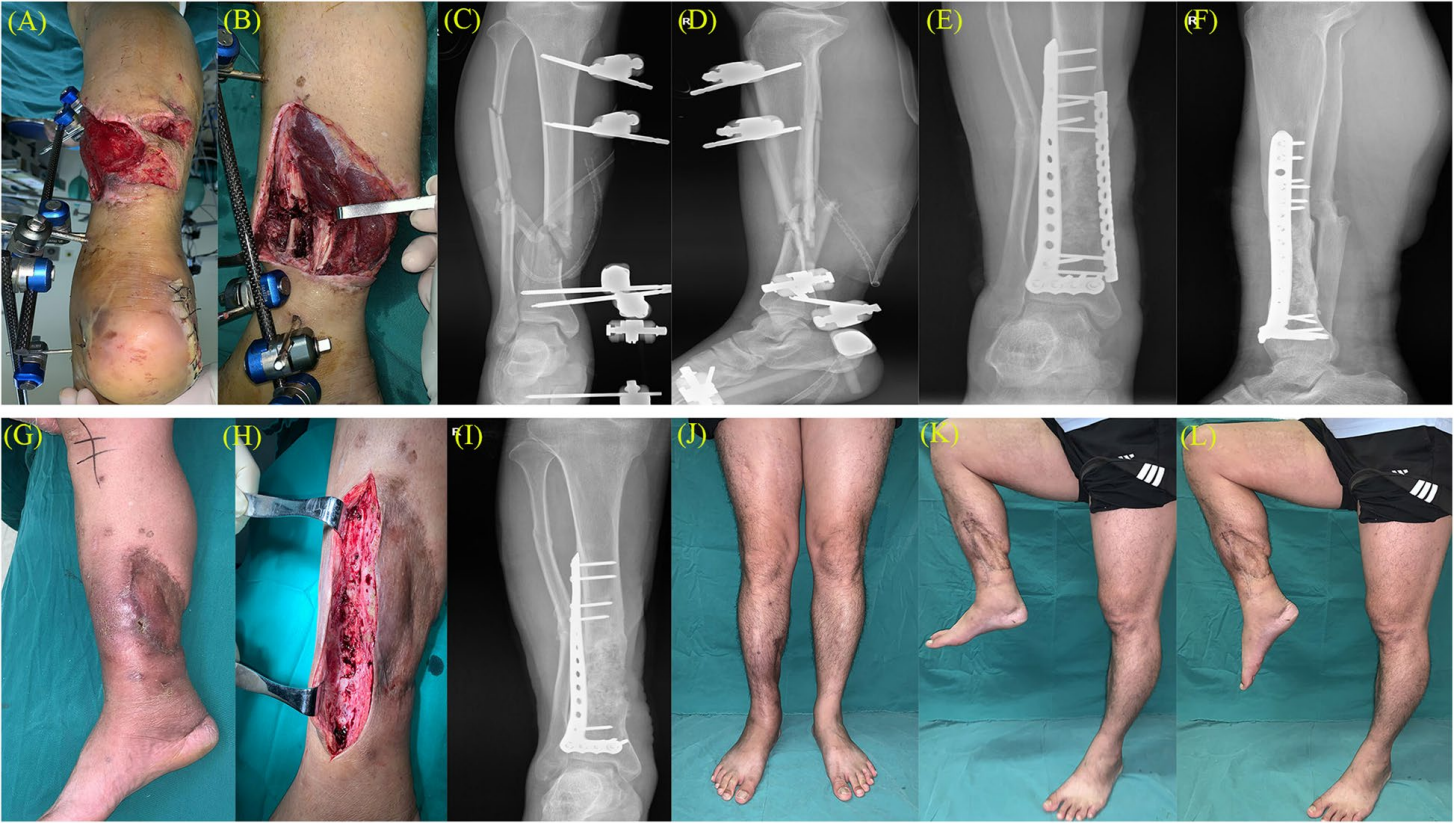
A/B/C/D Appearance of the affected limb and X-ray of the right calf when the patient is transferred from another hospital. E/F X-rays showed that the bone healed well at 13 months after bone grafting. G/H Redness, swelling and a small amount of exudation were found on the inner side of the right leg, and the plate on the inner side of the right leg was removed. I X-rays showed that no infection in the tibia at 3 months after removing the medial plate. J/K/L The Iowa ankle score was 86 at 6 months after removing the medial plate
